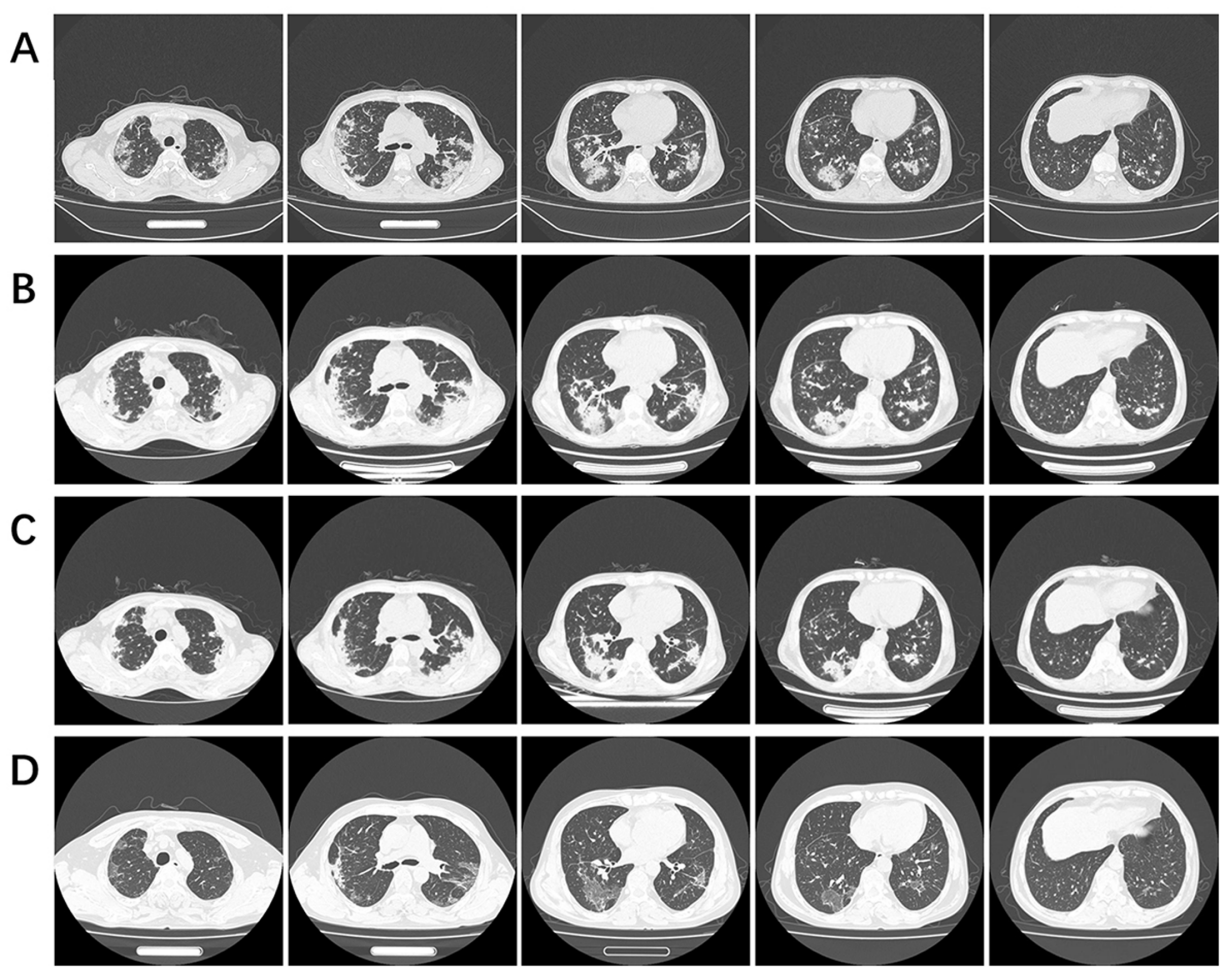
Chest CT images obtained after different treatment phases. (A) Upon admission; (B) after 7 days of treatment with meropenem (1 g q12h) and sulfamethoxazole (two tablets bid); (C) after 3 days of methylprednisolone (40 mg) treatment; (D) 2 months after sequential oral methylprednisolone treatment.
